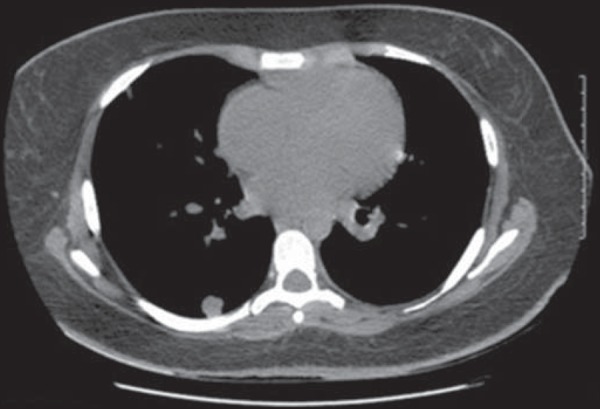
Contrast-enhanced CT of the chest, acquired during follow-up, showing
